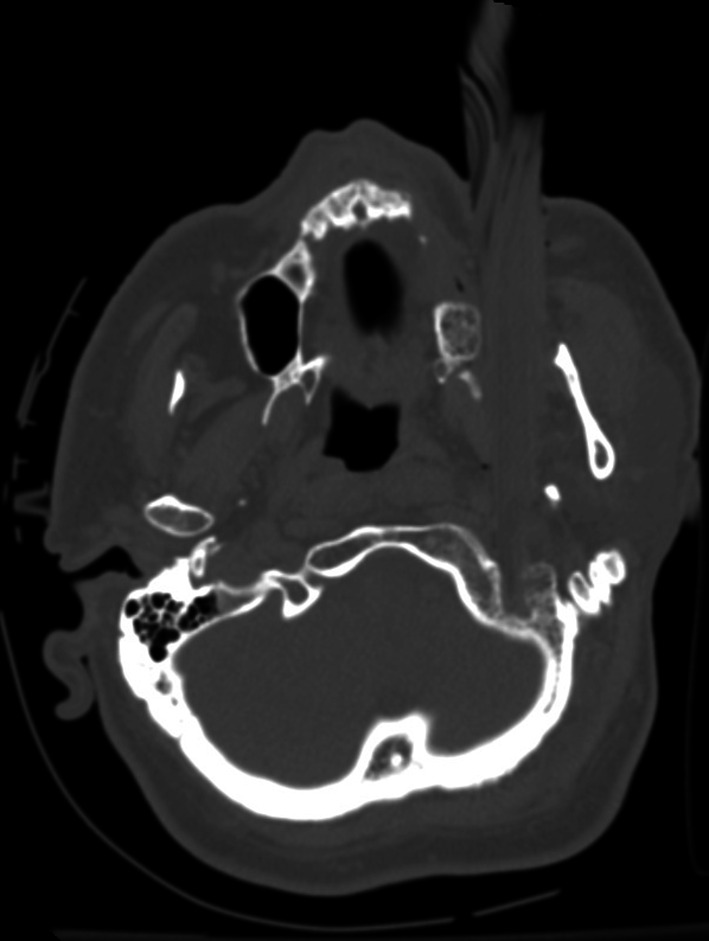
Case 1: localisation of the foreign body (CT)
